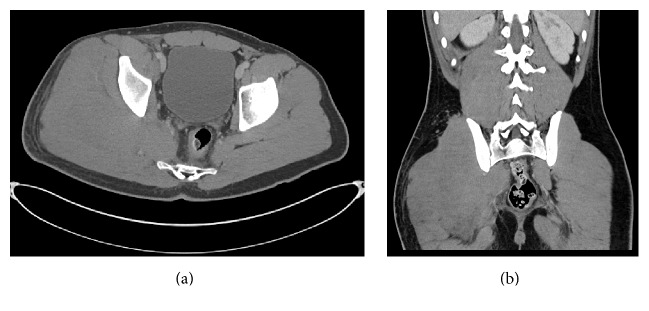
Axial (a) and coronal contrast enhanced CT (b) images of the abdomen and pelvis from the 17-year-old boy in case 1. There is striking asymmetric enlargement of the right gluteal and paraspinal muscles, isodense to adjacent muscle.
